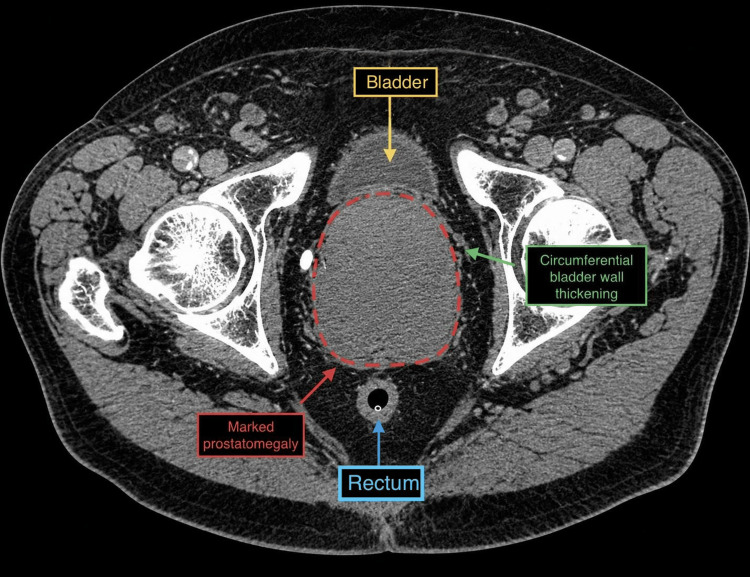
Axial contrast-enhanced CT Image demonstrating enlarged prostate and circumferential bladder wall thickening.
